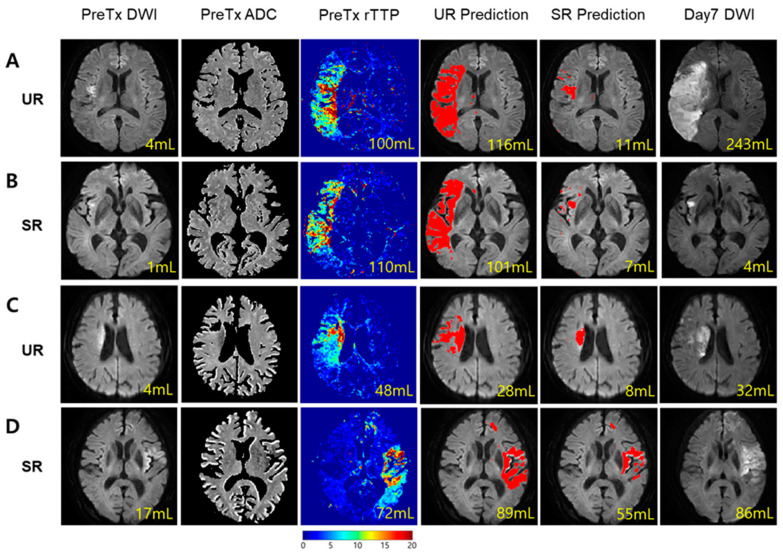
Sample external validation cases of SR- and UR-based infarct predictions. (A) A case of large infarct growth in a UR patient (intravenous thrombolysis, modified treatment in cerebral infarction (mTICI) = 0, and 90-day modified Rankin Scale (mRS) = 5). (B) A case of small infarct growth in an SR patient (endovascular therapy, mTICI = 2b, and 90-day mRS = 0). (C) A case of small infarct growth in a UR patient (endovascular therapy, mTICI = 1, and 90-day mRS = 2). (D) A case of large infarct growth in an SR patient (endovascular therapy, mTICI = 2b, and 90-day mRS = 5). Predicted infarct masks, shown in red, are overlaid on pre-treatment DWI images for UR and SR model predictions. All four patients had target mismatch presence but showed various degrees of infarct growth. The yellow numbers indicate the following volumes: (from left to right) baseline DWI lesion volume, baseline PWI lesion volume, UR-predicted infarct volume, SR-predicted infarct volume, and day-7 infarct volume.
